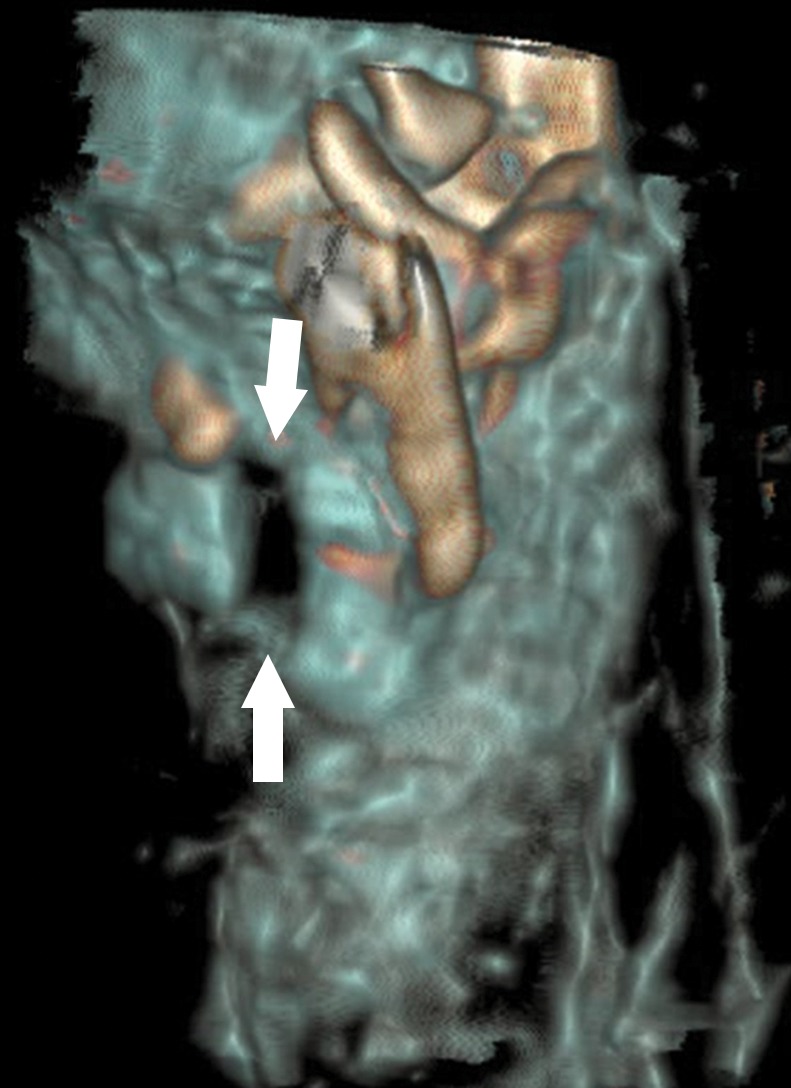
(a) Axial cervical postmortem CT scan and (b) zoomed section of the cricoid cartilage (box in a) of a 27-year-old woman who died of strangulation. Three-dimensional volume-rendered reconstructions from cranial (c), left lateral oblique (d), and right lateral oblique (e) views. Postmortem CT scan learly displays a displaced bilateral fracture of the cricoid cartilage (arrows). This finding is important because it proves the application of relevant force to the neck. It was difficult to demonstrate this finding at autopsy because anatomic preparation required extensive manipulation of the laryngotracheal region, which without postmortem CT would have been unclear regarding whether the fracture was caused by the preparation or was there before autopsy.
